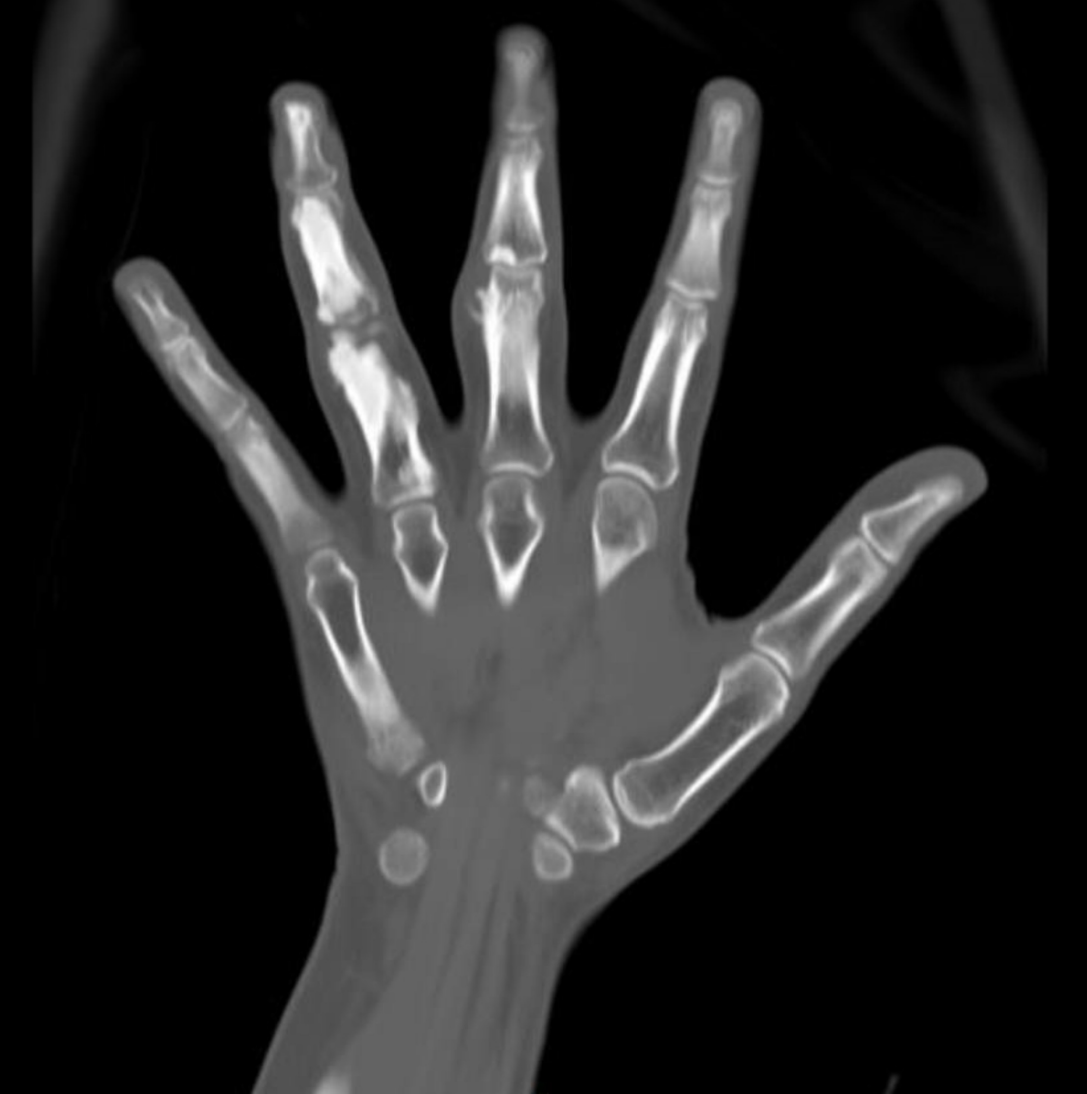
CT scan of the right hand.
